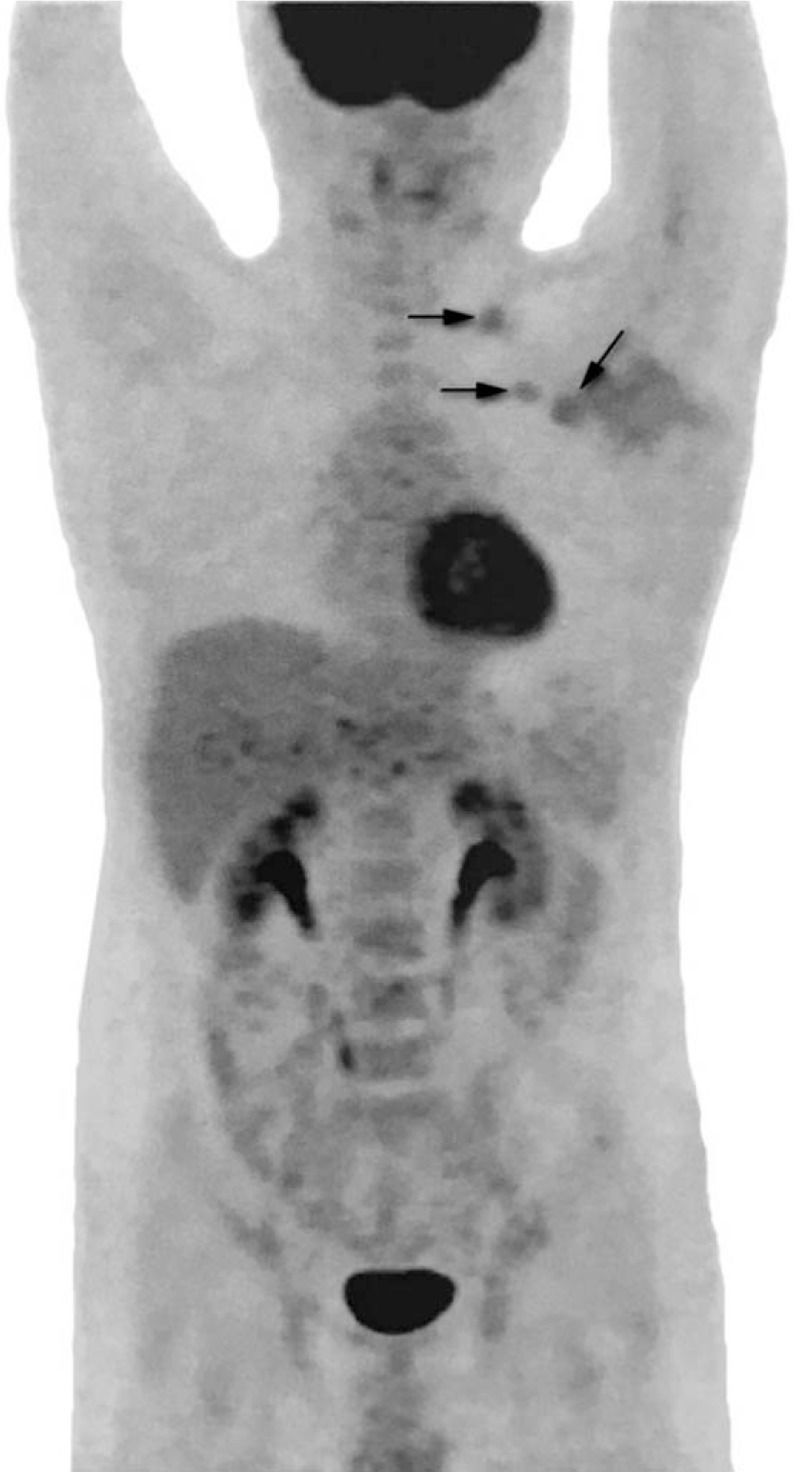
PET/CT scan of the entire body showed several lesions with increased uptake in the left axillary and infraclavicular regions (arrows) after the first surgery. PET/CT = positron emission tomography/computed tomography.
